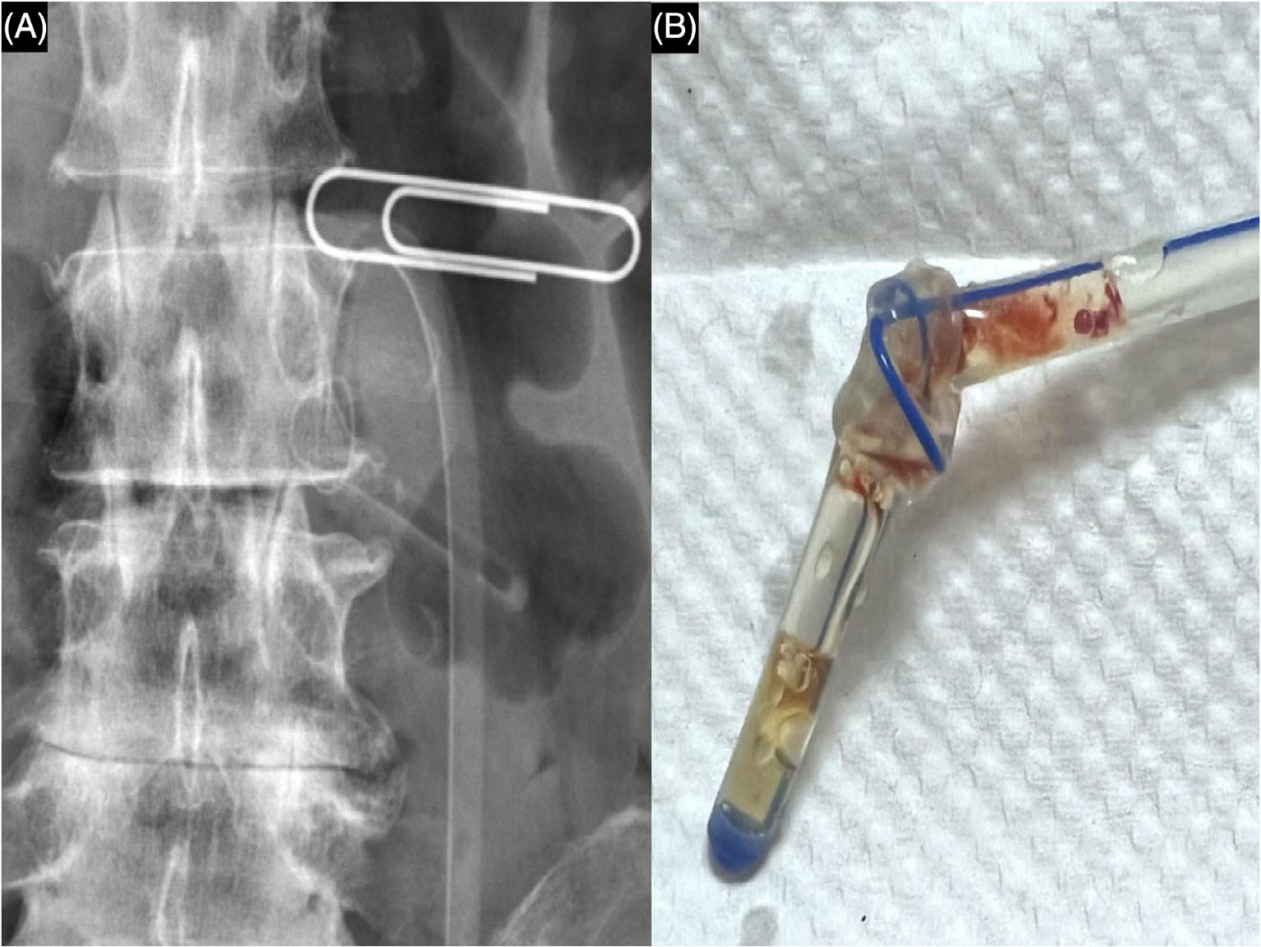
(A) The abdominal radiograph revealed knotting and kinking of the feeding jejunostomy tube. (B) Self‐knotted feeding jejunostomy tube was directly removed without additional invasive procedures.
